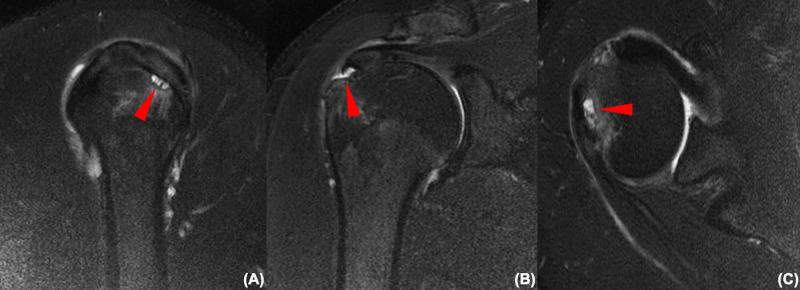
MRI images (A: sagittal view; B: coronal view; C: axial view) showing an obvious supraspinatus full-thickness tear as well as an increased signal inferi1or to the full-thickness tear (red arrows).
